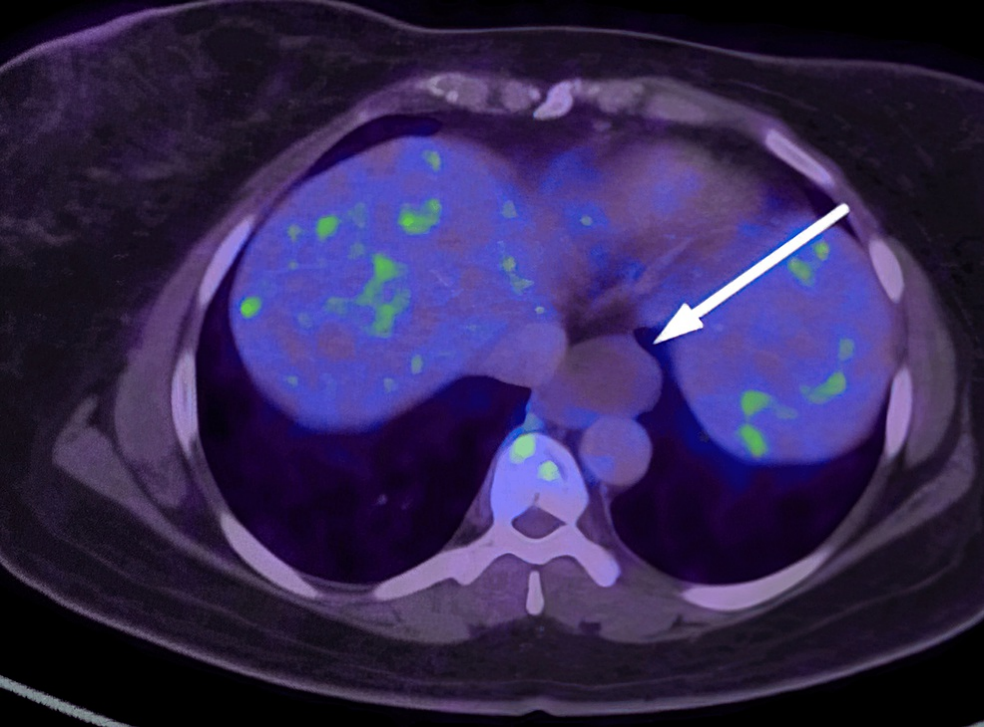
Non-FDG avid non-enhancing lesion (40 x 27 x 35 mm, SUV max 1.05) seen along the left lateral wall of the distal esophagus (white arrow)
